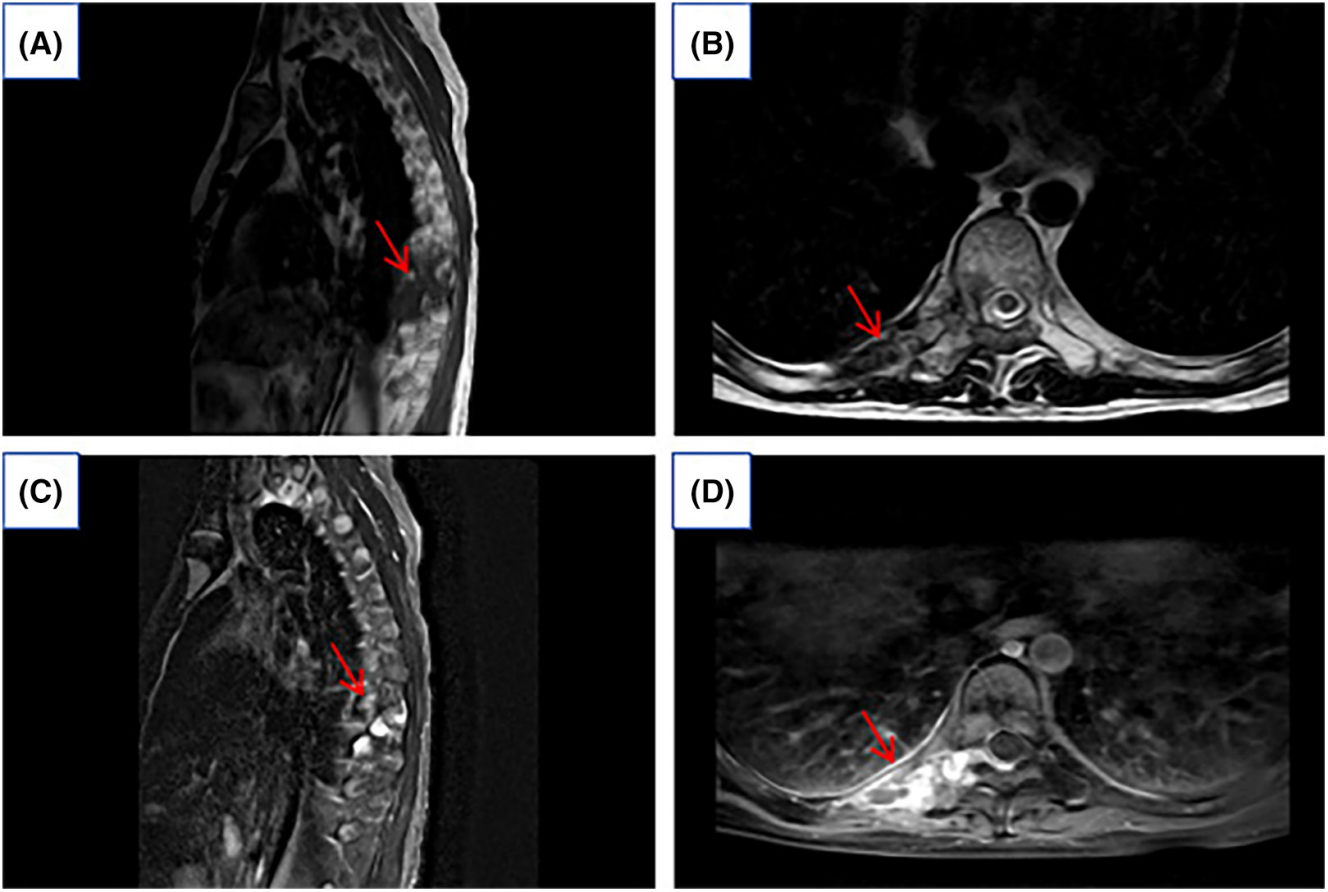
Preoperative MRI scanning with enhancement shows a mass‐like mixed signal shadow measuring approximately 2.42 cm × 1.37 cm. (A) The lesion was isosignal in the sagittal position of T1WI. (B) T2WI transects showed mixed high and low signals with predominantly high signals. (C) T2WI sagittal pressure lipids showed iso‐hypermixed signals with predominantly high signals. (D) T1WI transverse enhancement scan of the lesion showed marked inhomogeneous enhancement, within which streaks of unenhanced areas were observed (red arrows in A, B, C, and D).
